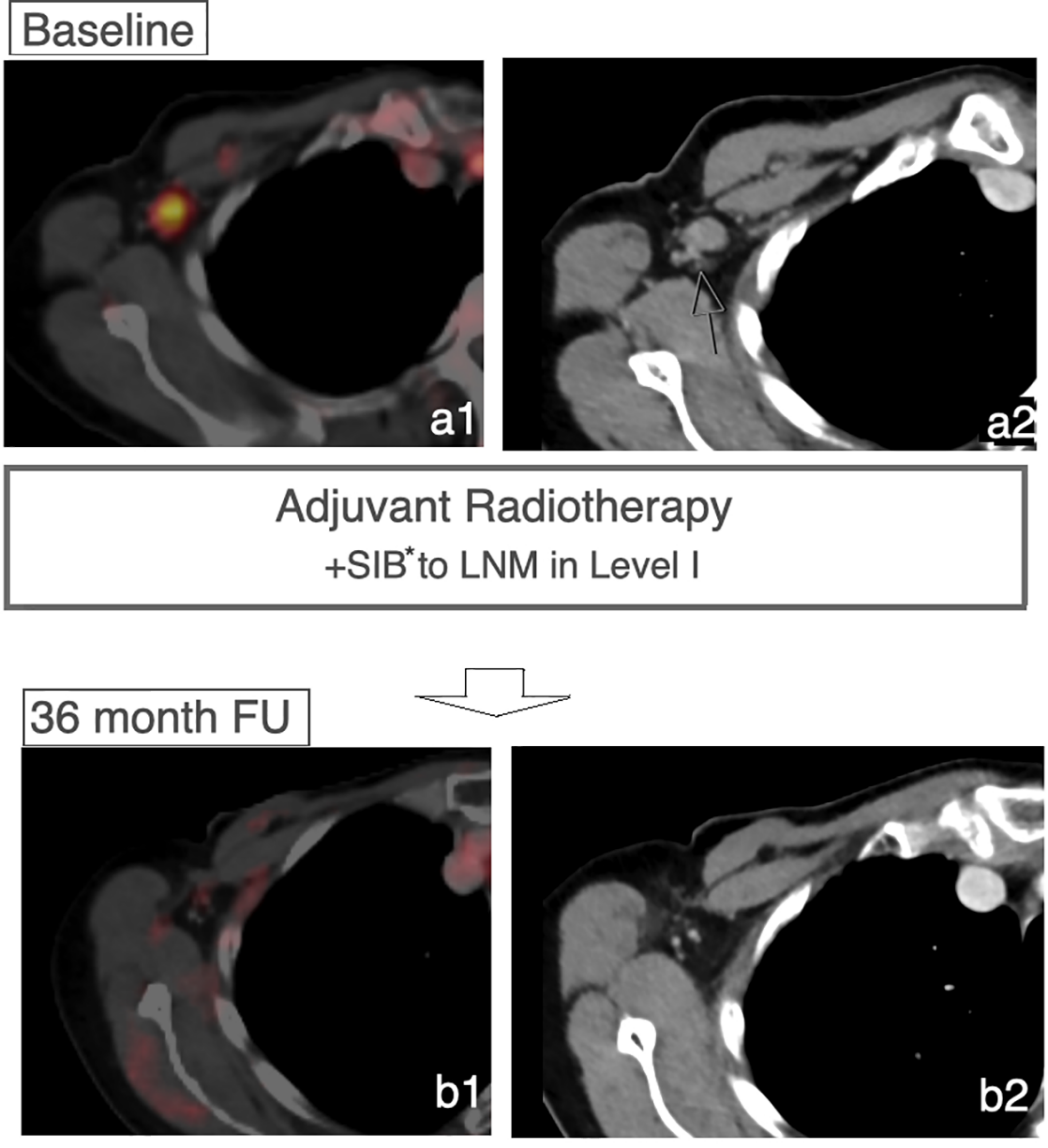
Controlled LN after LN-SIB. Case of a patient receiving a LN-SIB to a remaining sLN in Level I. FDG-PET/CT imaging after 36 months shows regression without signs of recurrence within the lymphatic drainage system. *Dose 58.8 Gy in 28 Fx.
